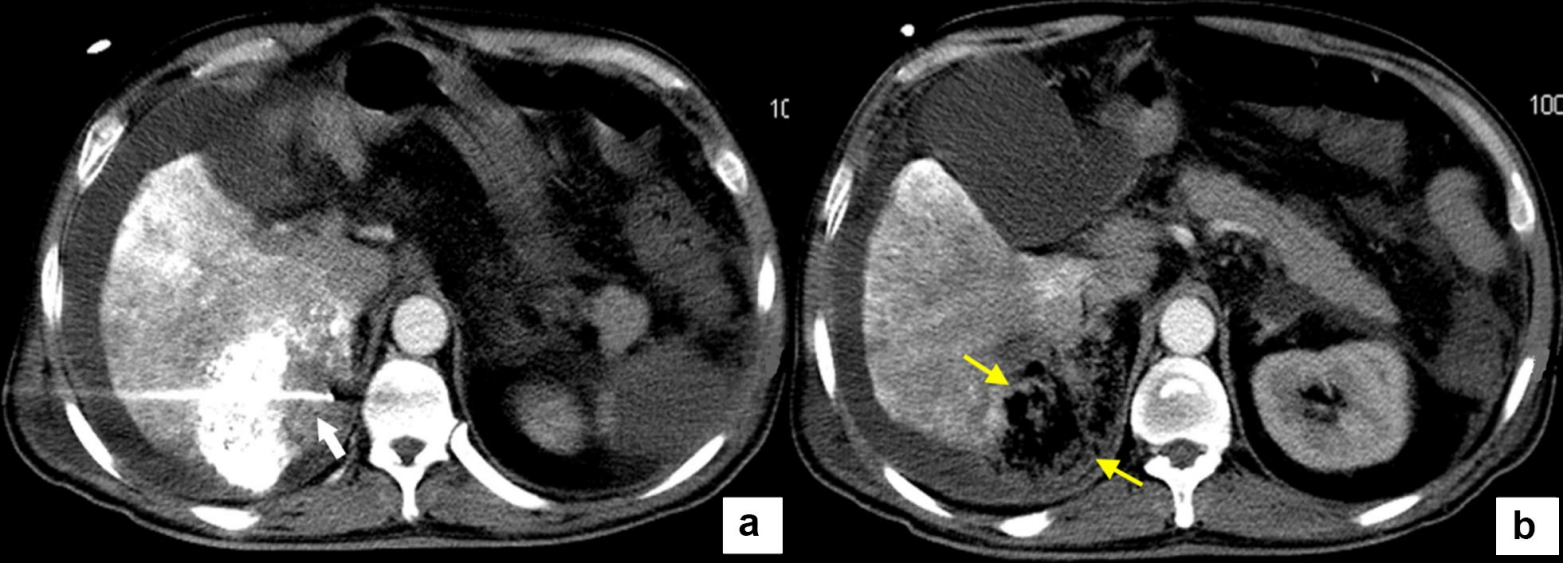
The CT images of the hydrochloric acid (HCl)-enhanced radiofrequency ablation (RFA) procedure. a The RFA electrode (*arrow*) is inserted into the portion without lipiodol deposition. b The loose necrotic HCC lesion after HCl-enhanced RFA procedure hangs on the renal region (*twin arrows*)
